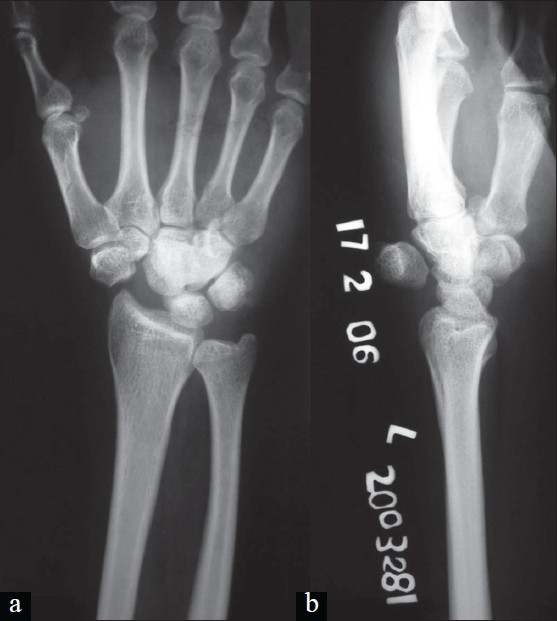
(a) Anteroposterior radiograph of left wrist shows missing scaphoid and loss of Gilula arc. (b) Lateral radiograph showing dorsally dislocated scaphoid.
